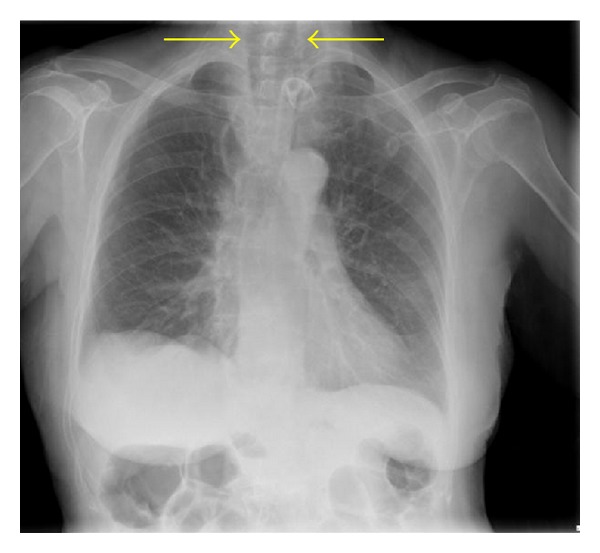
Chest radiograph showing large air shadow in the neck.
